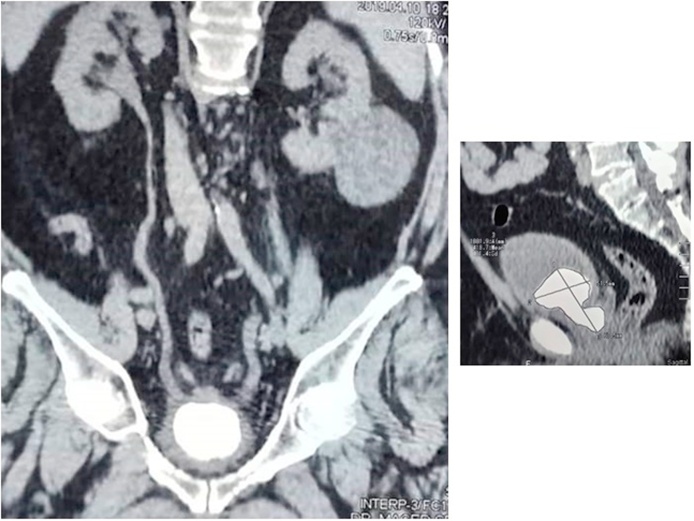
CT image of the prostatic cavity large stone measuring 3.2 × 2.8 cm.
